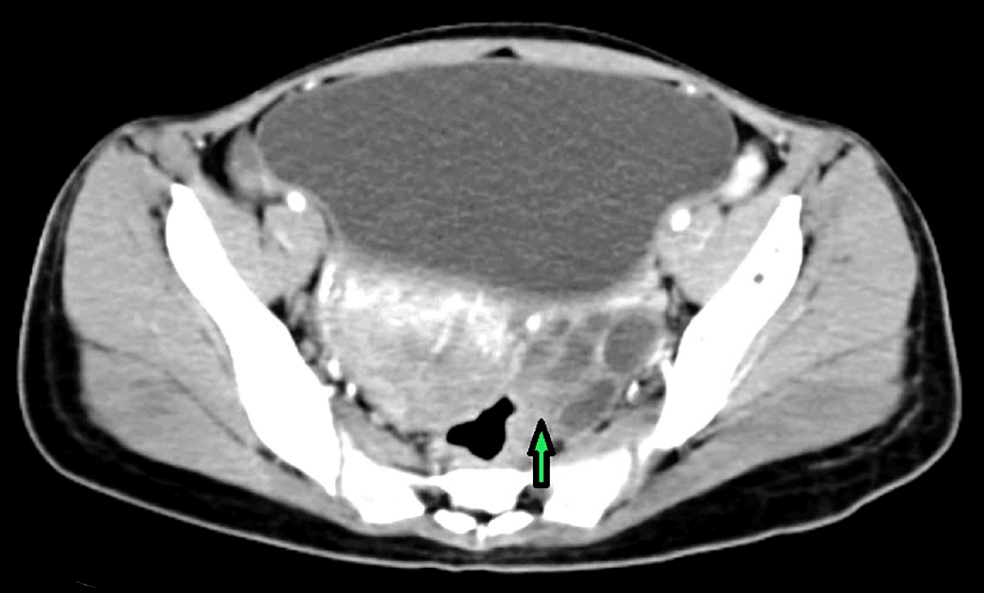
HRCT showing multiple cystic lesions in the ovary (arrow) HRCT: High-resolution computed tomography
